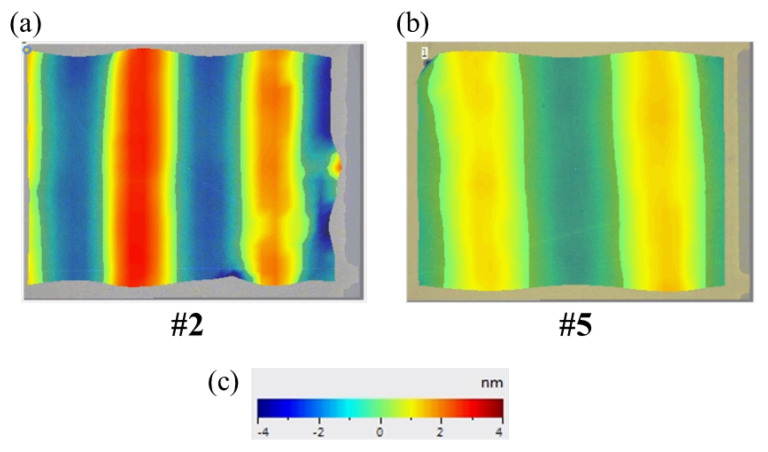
Lamb waves characterization using laser Doppler vibrometry (LDV) when the input power is 0.65 W. (a) The #2 Lamb wave transducers case (h/λ≈ 1.25), (b) the #5 Lamb wave transducers case (h/λ≈ 0.75), and (c) the amplitude corresponding to the scale bar from −4 nm to 4 nm. All LDV views denote a 800 μm length and a 600 μm width.
